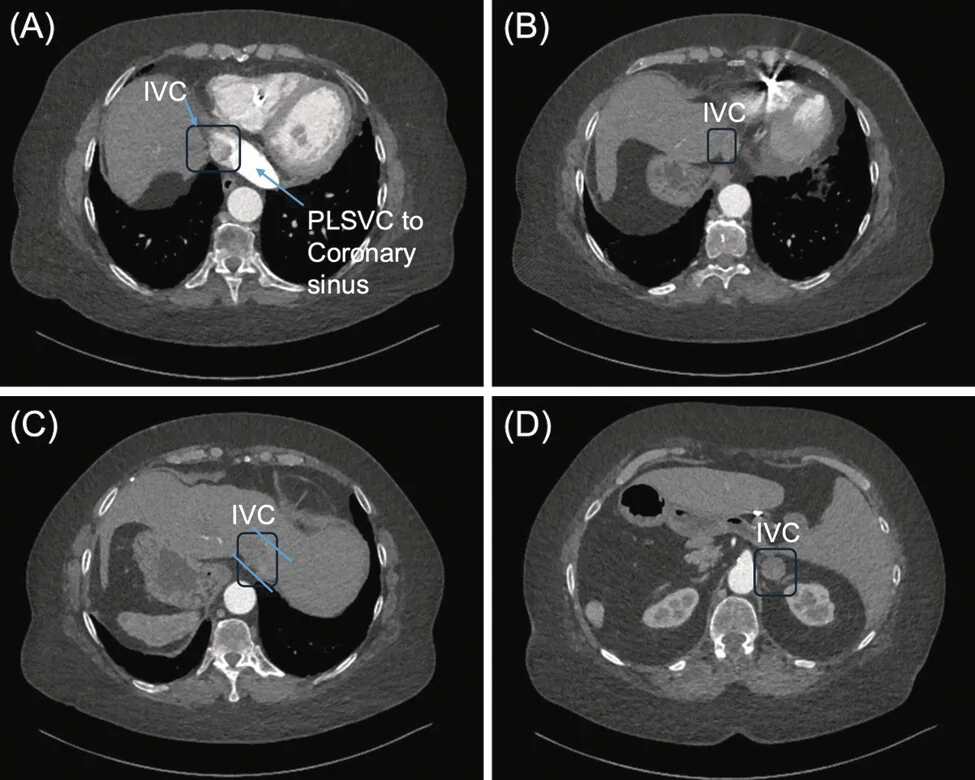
Axial CT sequence demonstrating the tortuous course of the left-sided IVC. A series of four axial contrast-enhanced CT slices from the midcardiac level (upper panels) to the hepatic level (lower panels).
